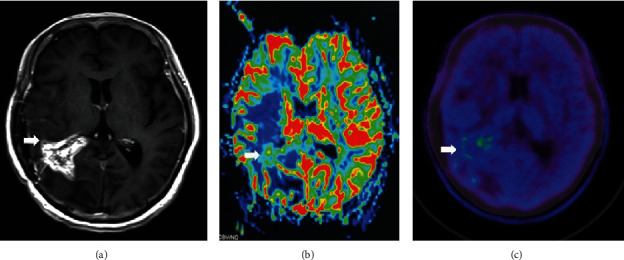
MET PET with PWI of radiation necrosis. Contrast-enhanced T1-weighted MR image (a) and relative CBV map (b) and MET PET/CT image (c) for a 44-year-old man with necrosis. The lesion in the right parietal lobe (arrow) shows enhancement (a) and negative findings on both the rCBV map (b) and the MET PET/CT image (c). Reproduced with permission from Figure 3 of Qiao et al. [58].
